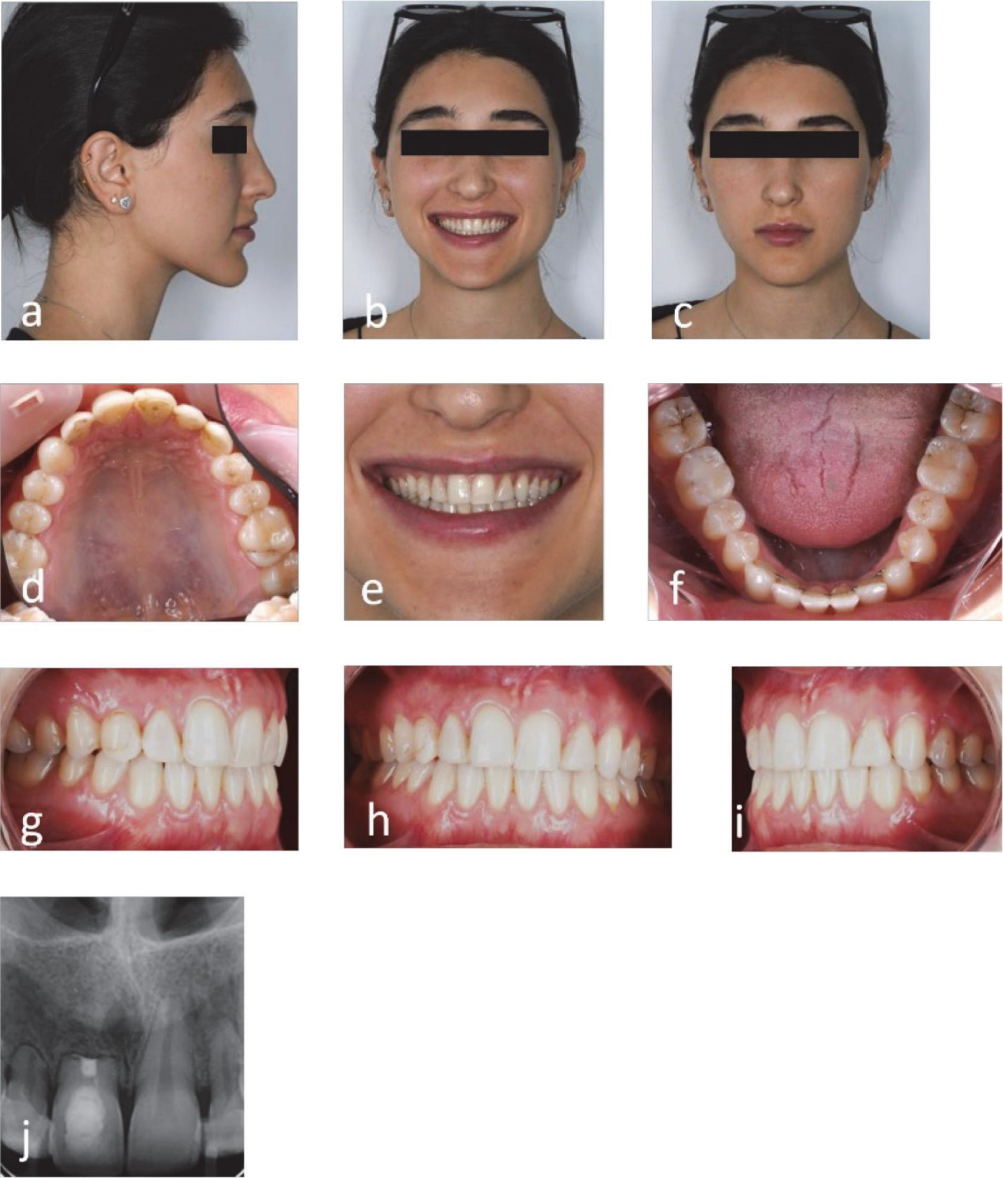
Follow‐up photographs and radiographs 15 years after endodontic microsurgery: (a–c) extraoral view with harmonious facial esthetics and smile; (d–i) intraoral view with aligned arches and Class I occlusion; and (j) periapical radiograph showing stability of the maxillary right incisor.
